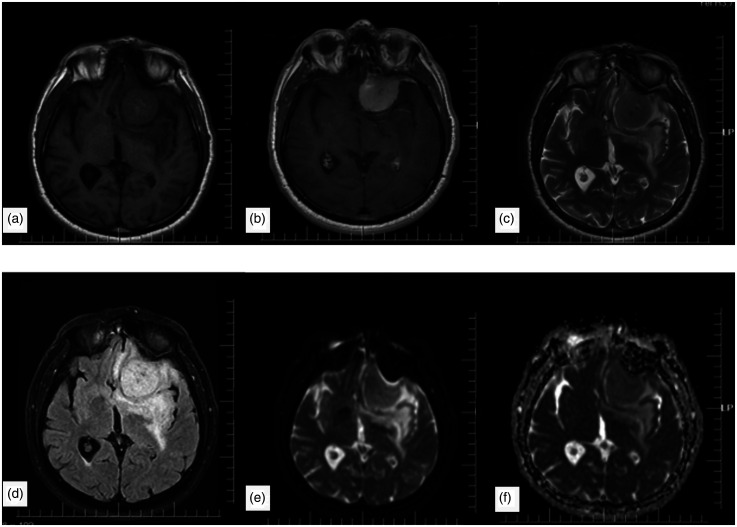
A meningioma case in the left frontal region. Hypo-isointense on (a) T1-weighted and (c) T2-weighted images, (b) intensely enhancing after contrast administration, and (d) hyperintense on FLAIR images. There is mild peripheral edema around the tumor. (e) DWI and (f) ADC maps show no significant diffusion restriction. ADC, apparent diffusion coefficient; DWI, diffusion-weighted imaging; FLAIR, fluid-attenuated inversion recovery.
